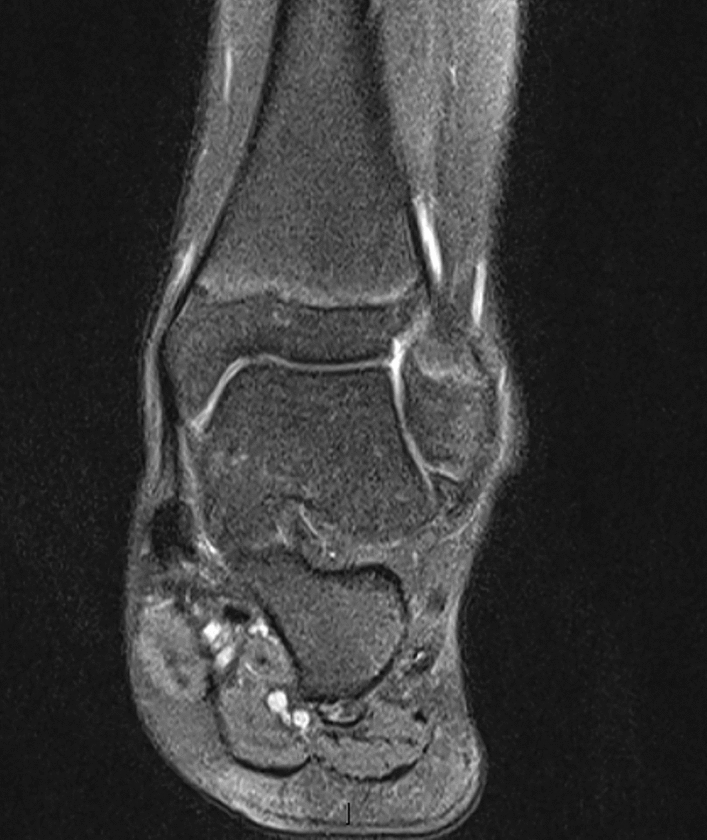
MRI scan of the ankle with os subfibulare-ligamentous complex
